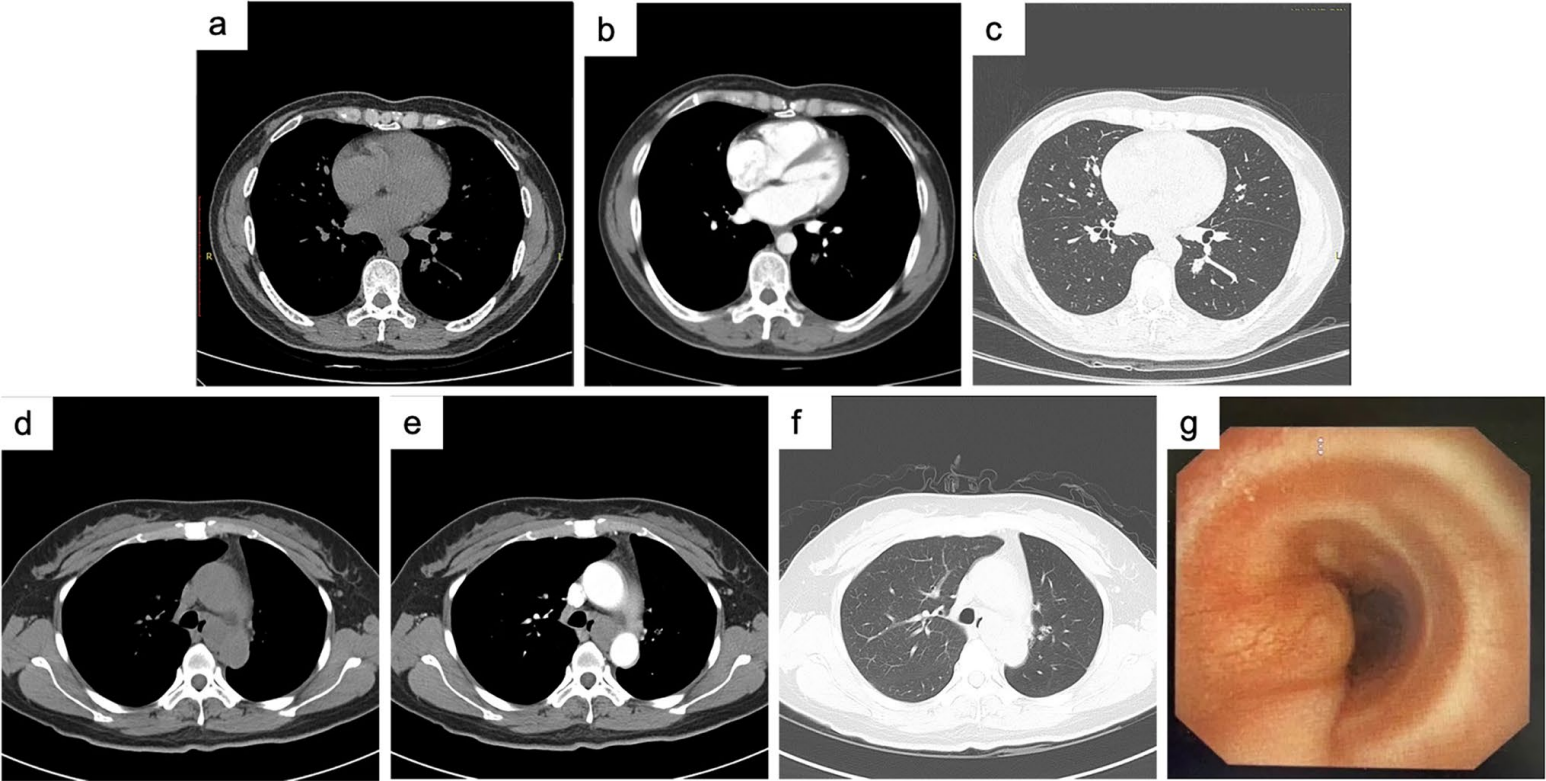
CT and bronchoscopy images of pulmonary adenoid cystic carcinoma of peripheral type a–c and central type d–g. a, d Plain transverse image of mediastinal window; b, e Enhanced transverse image of mediastinal window; c, f Transverse image of the lung window; g Bronchoscopy images
